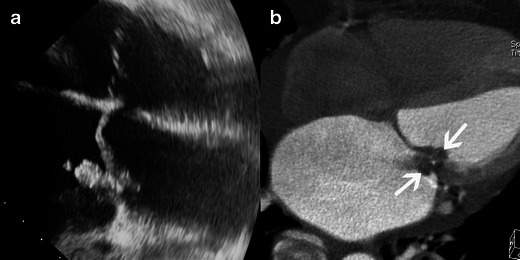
Results of echocardiography and MSCT studies in cases of mitral valve IE. Images show four-chamber views in the TEE study (a) and four-chamber view MSCT acquisitions with MPR reconstruction (b). Both TEE and MSCT show a large vegetation (*white arrow*) and destruction of the mitral valve with substantial dilatation of the left atrium and pericardial effusion
